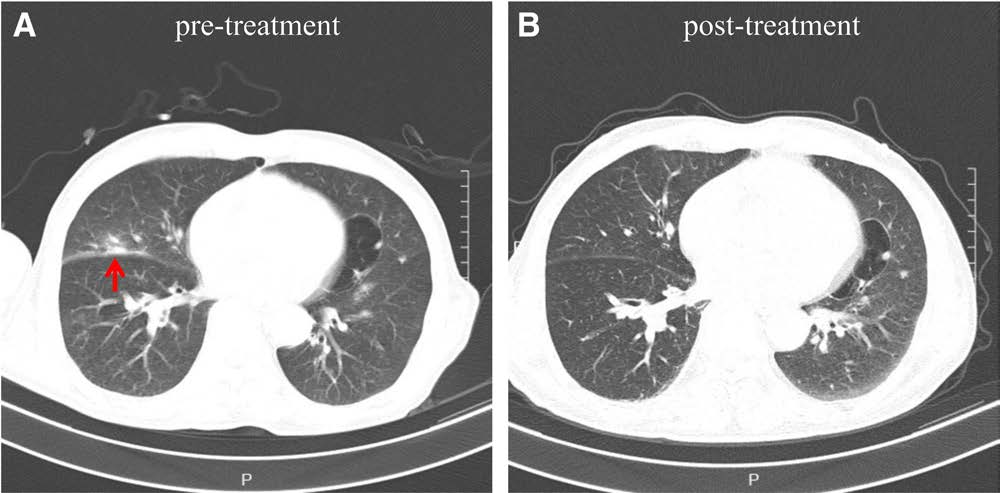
Chest CT scans during anti-infective therapy. (A) Radiographic abnormalities before antimicrobial treatment showed the inflammatory foci were noted in the left lung (red arrowhead). (B) Radiographic imaging after 3 months of treatment with minocycline, showed the inflammatory foci in the left lung was gone.
